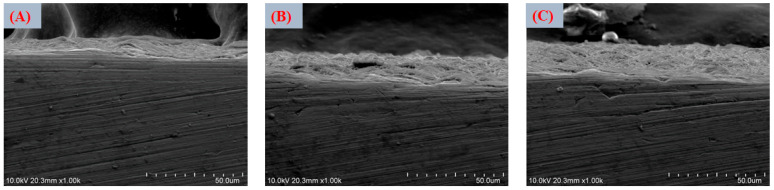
FESEM images of the adhesion stability of Zn-CaP variants after mechanical handling and ultrasonic cleaning. (A) VDZn1, 1000×, (B) VDZn2, 1000×, and (C) VDZn3, 1000×.
